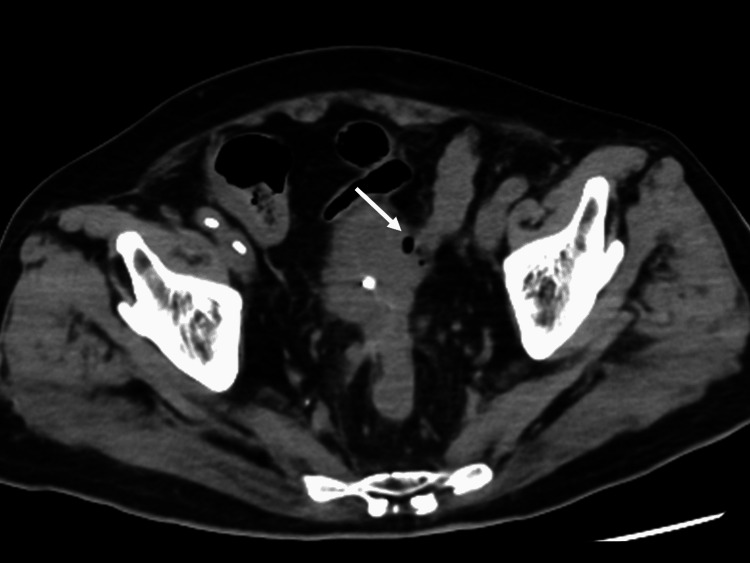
Plain pelvic CT on arrival Pelvic CT showed small amounts of gas at the bladder wall where it contacted the sigmoid colon.
